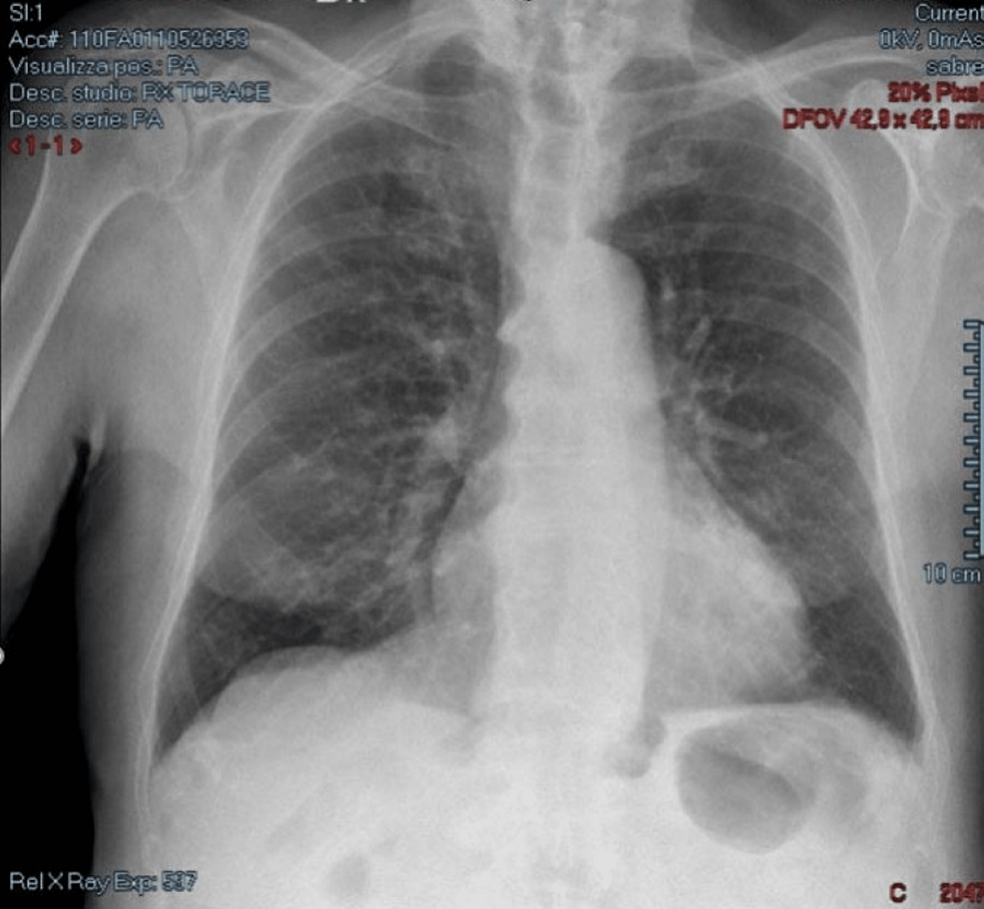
Chest X-ray showing interstitial pneumonia
